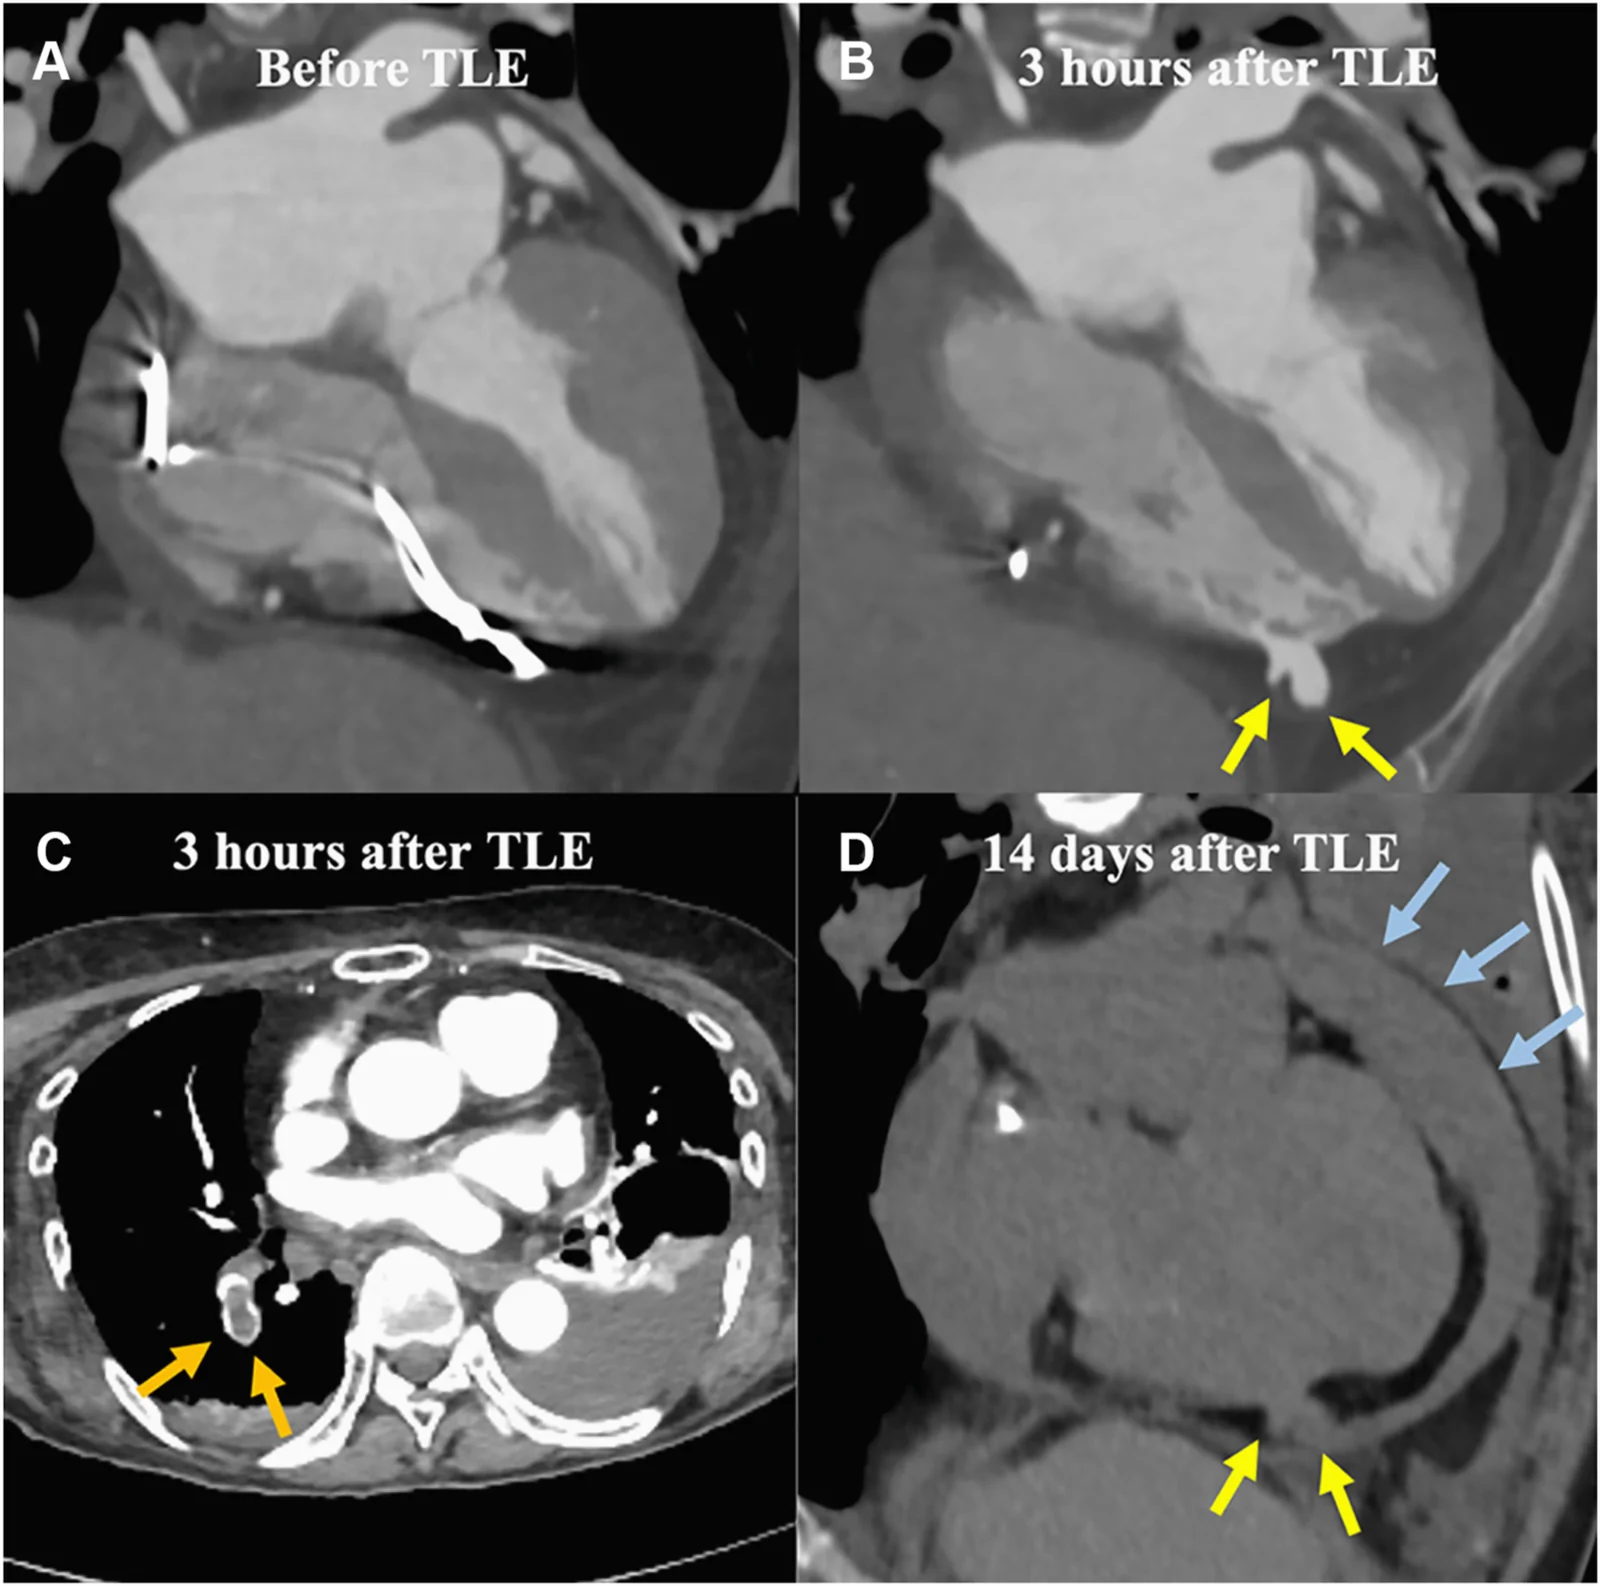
Serial oblique and axial computed tomography images. A: On admission (preoperative). B: 3 hours after TLE. C: An axial computed tomography obtained 3 hours after TLE. D: Day 14 after TLE. (*Blue arrows*) Pericardial effusion. (*Yellow arrows*) RV pseudoaneurysm. (*Orange arrows*) Contrast filling defects in branches of the right pulmonary artery. RV = right ventricle; TLE = transvenous lead extraction.
